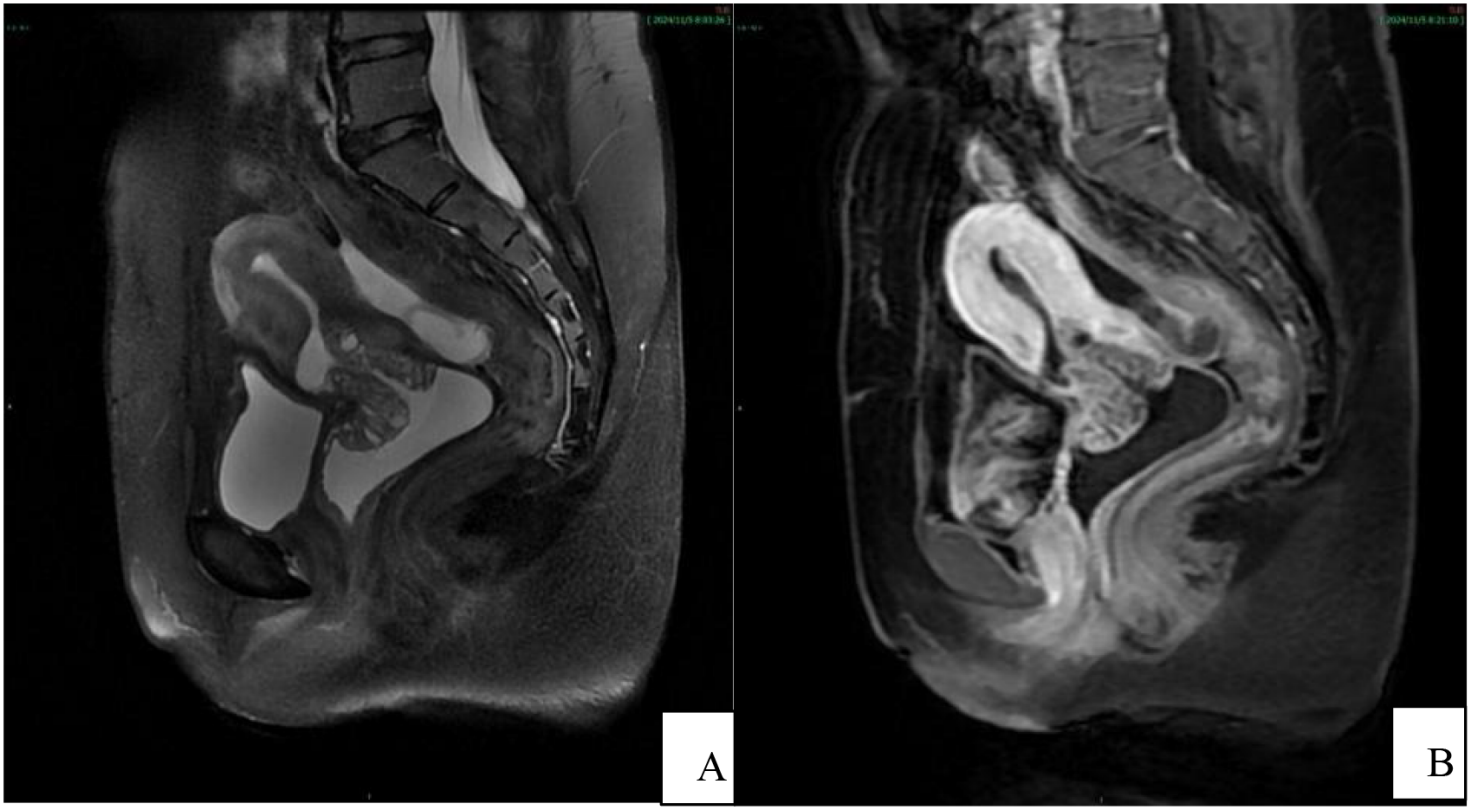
Pelvic MRI sagittal position. (A) T2WI shows an enlarged cervix with diffuse, irregularly sized, quasi-circular or small patchy high signal intensity shadows on the cervix wall, with unclear boundaries and some visible fine septa within; the image reveals intrauterine fluid accumulation and post-cesarean section changes on the anterior wall of the uterus; (B) In the arterial phase of enhancement, the cervical lesion exhibits uneven enhancement, with significant enhancement of the cyst wall and no obvious enhancement within the cyst. The lesion involves the lower 1/3 of the uterine body and the upper 1/3 of the anterior wall of the vagina, with unclear demarcation between the lesion and the posterior wall of the bladder.
